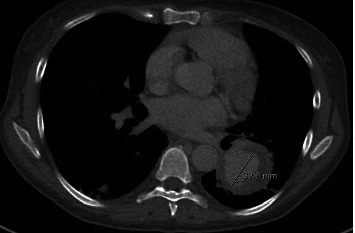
Axial computed tomography angiography depicting a pseudoaneurysm measuring 52 × 47 mm with an irregular central contrast collection measuring 32 mm (marked above).
